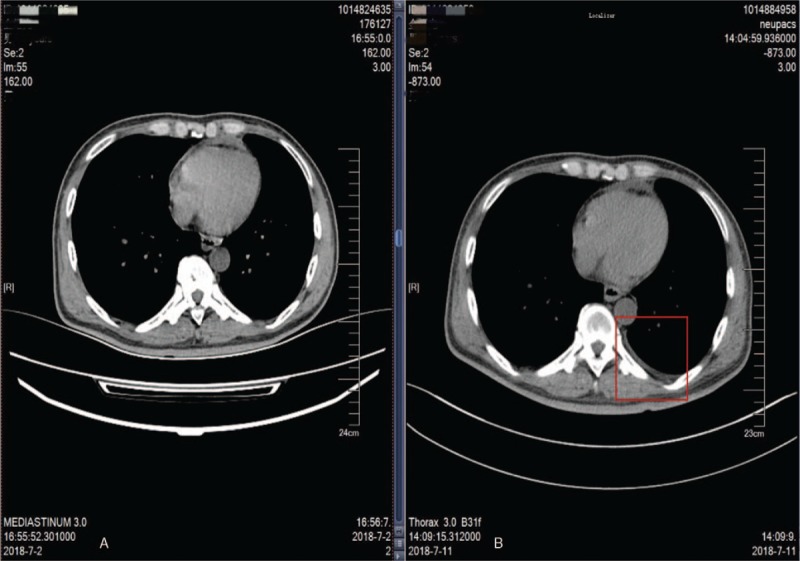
Chest CT of different periods. (A) Chest CT before RPE. (B) Chest CT after RPE. CT = computed tomography, RPE = re-expansion pulmonary edema.
